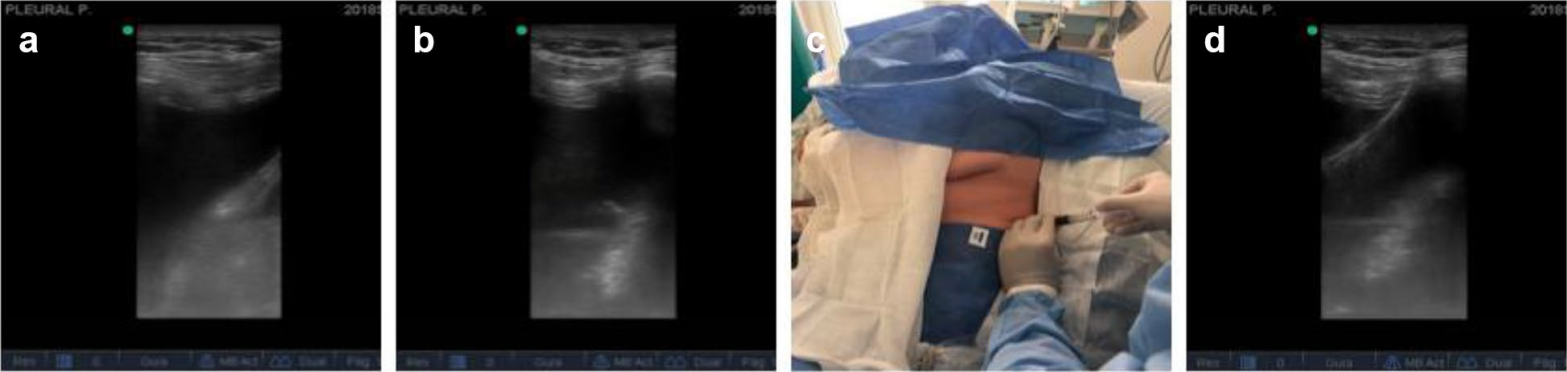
a Assessment, with a 13-MHz linear transducer, of the effusion in the lower aspect of the thorax on the posterior axillary line, visualizing the upper and lower ribs, intercostal space, pleural fluid, and diaphragm. b After verifying no interposition of vessels with color Doppler, needle puncture is performed; needle passage into the pleural cavity is visualized in real time. c Pleural fluid return is verified, in this case blood. d Passage of the guide toward the pleural cavity on the diaphragm
